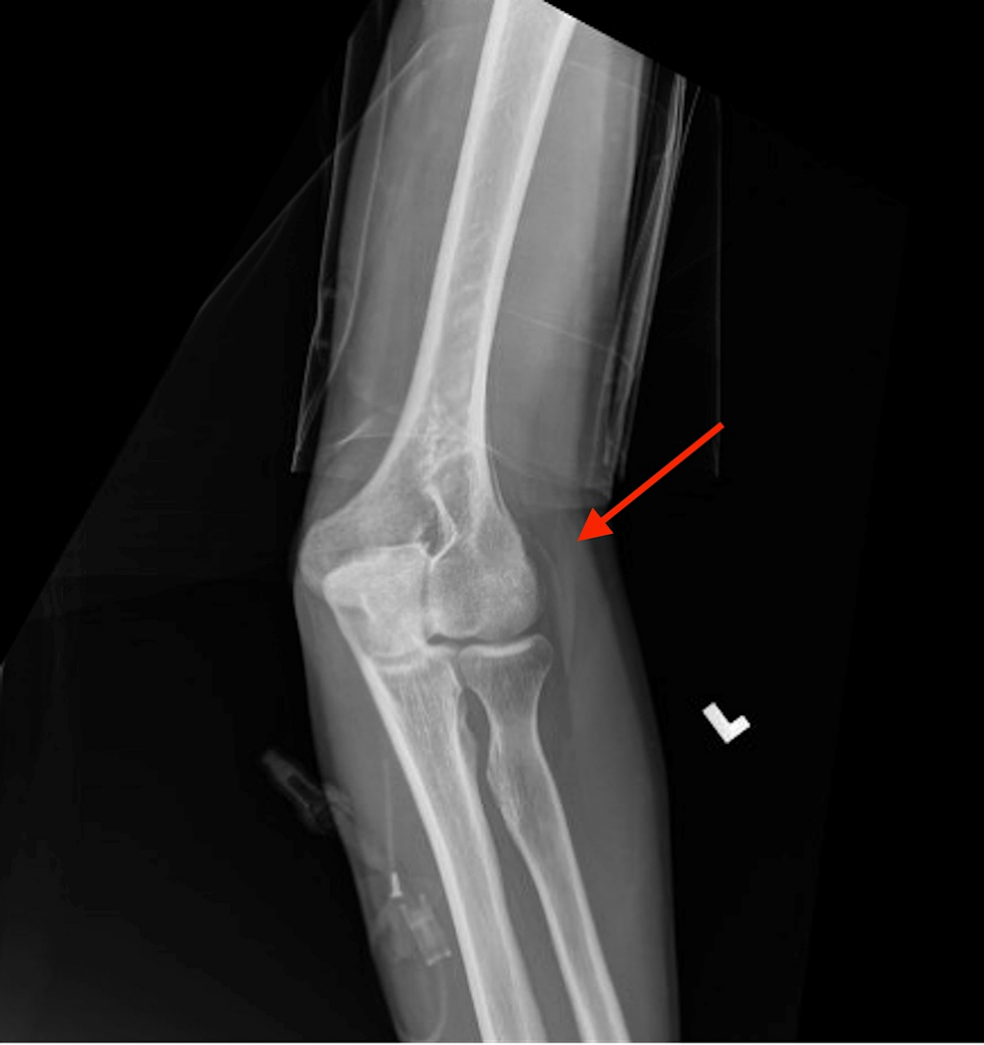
X-ray of the left elbow revealing joint effusion (red arrow)
